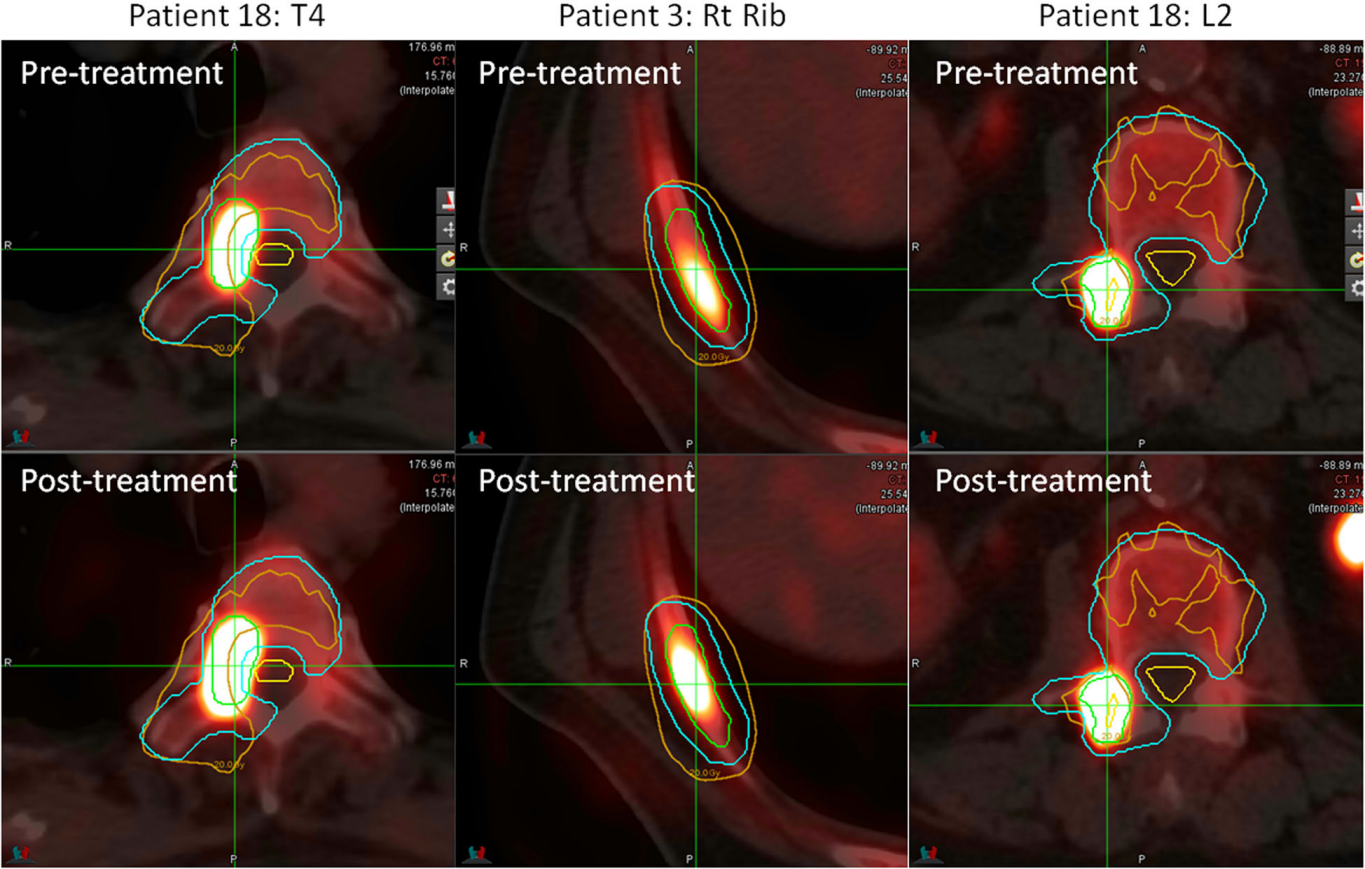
Pre and post-treatment NaF scans for the two patients (three lesions) with increased SUV_max_ in the tumour. The tumour is shown by the green contour, the PTV in blue and the volume receiving 20 Gy in orange. The spinal cord is shown in yellow for Patient 18
